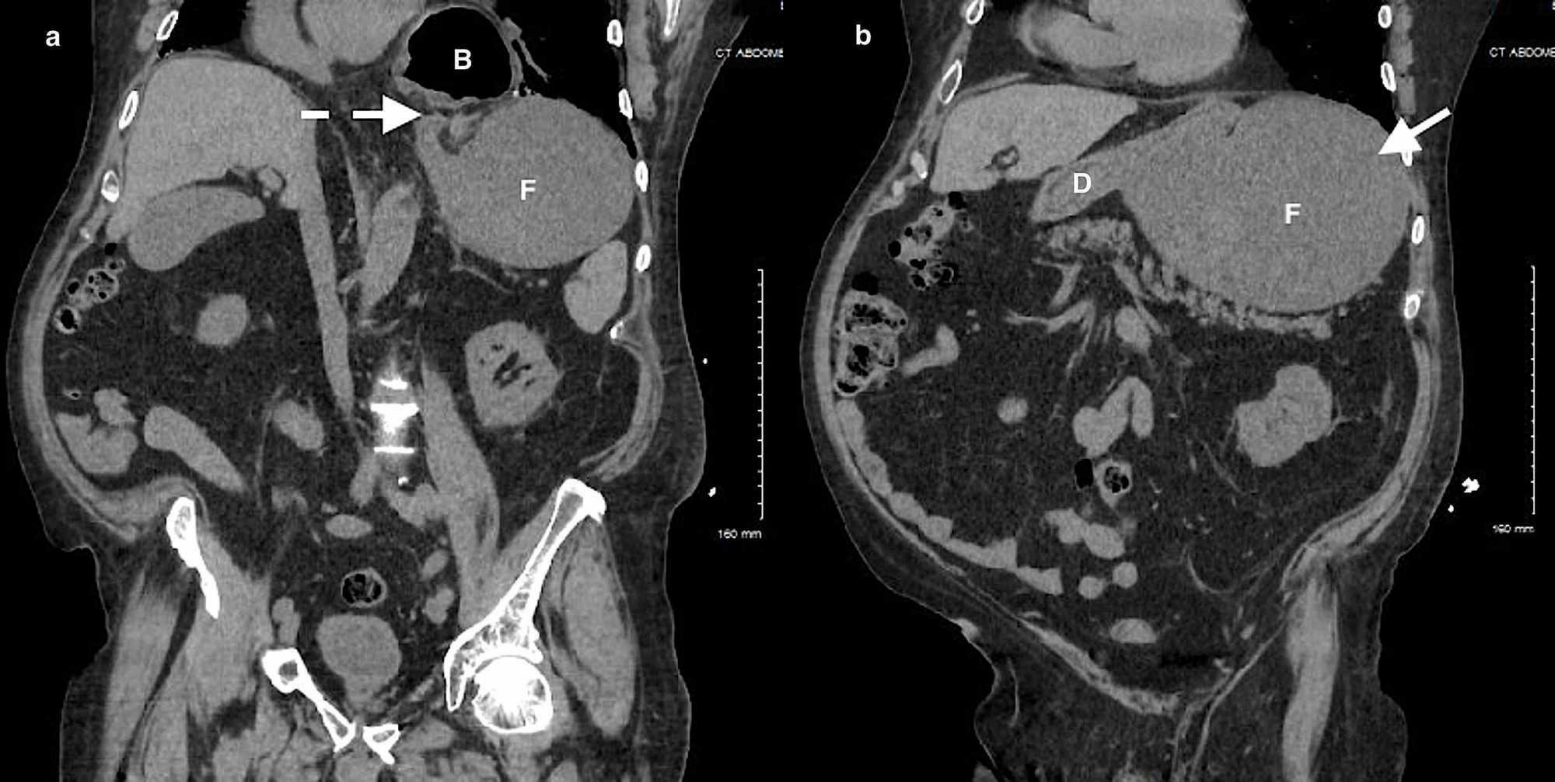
CT of the abdomen/pelvis (coronal views) demonstrating gastric volvulus with (a) malrotation (dotted arrow) and a (b) fluid-filled distended stomach (solid arrow). B, gastric body; F, gastric fundus; D, duodenum.
